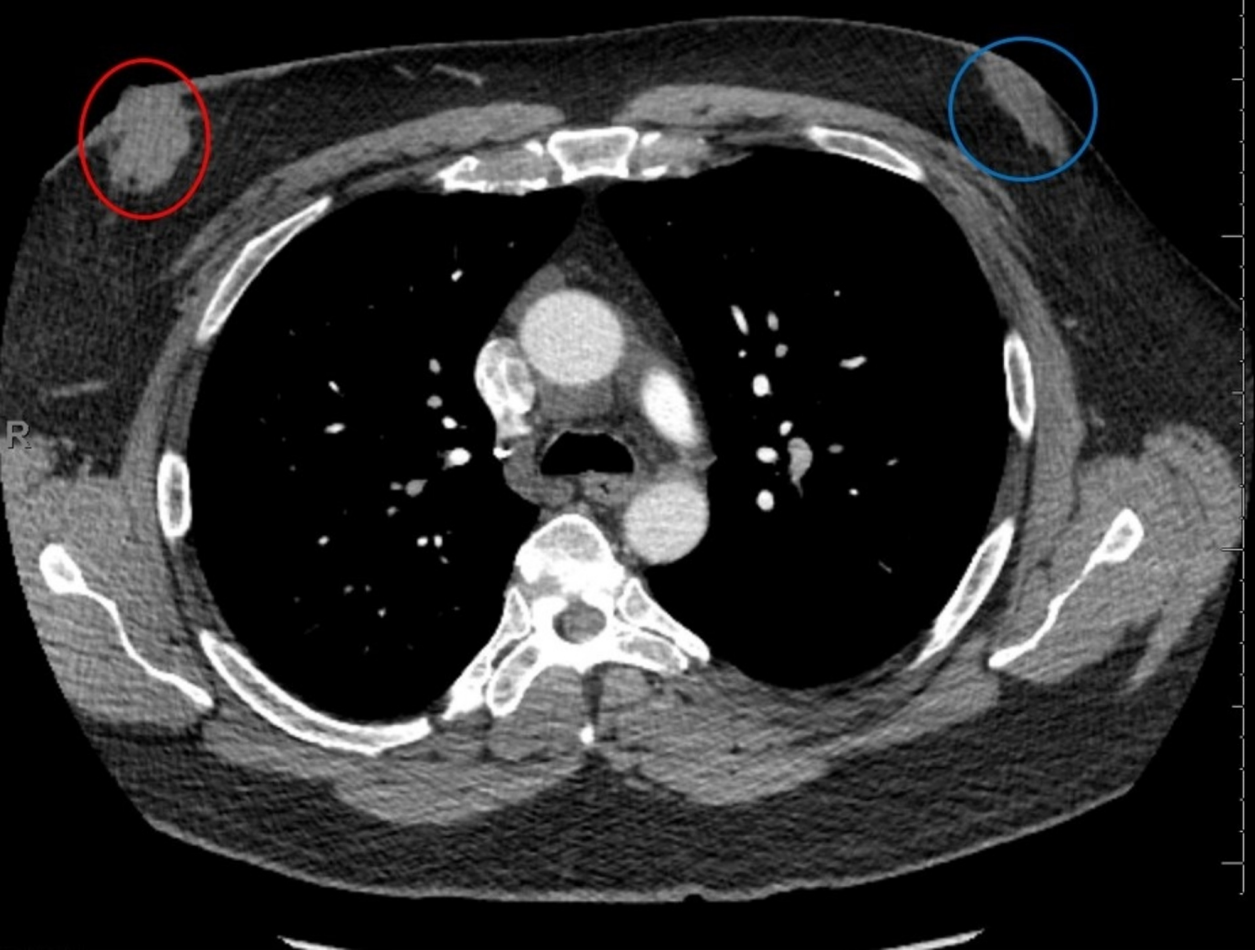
CT chest Right breast biopsy-proven malignancy (red circle); left breast gynecomastia (blue circle); no evidence of intra-thoracic metastases. CT, computed tomography
